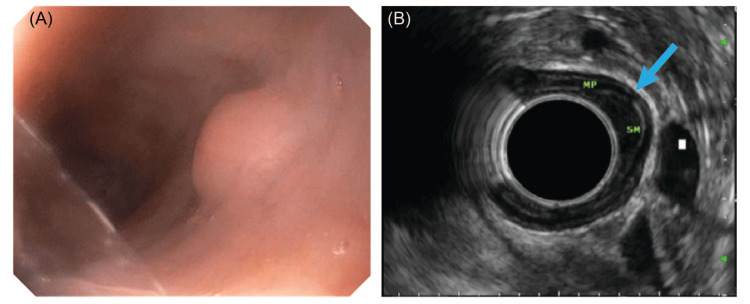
(A) EGD showing a raised submucosal nodule in the middle third of the esophagus with a smooth surface. (B) EUS reveals a submucosal oval 8 mm × 3 mm intramural hypoechoic lesion (marked by the blue arrow). EGD, esophagogastroduodenoscopy; EUS, endoscopic ultrasound
